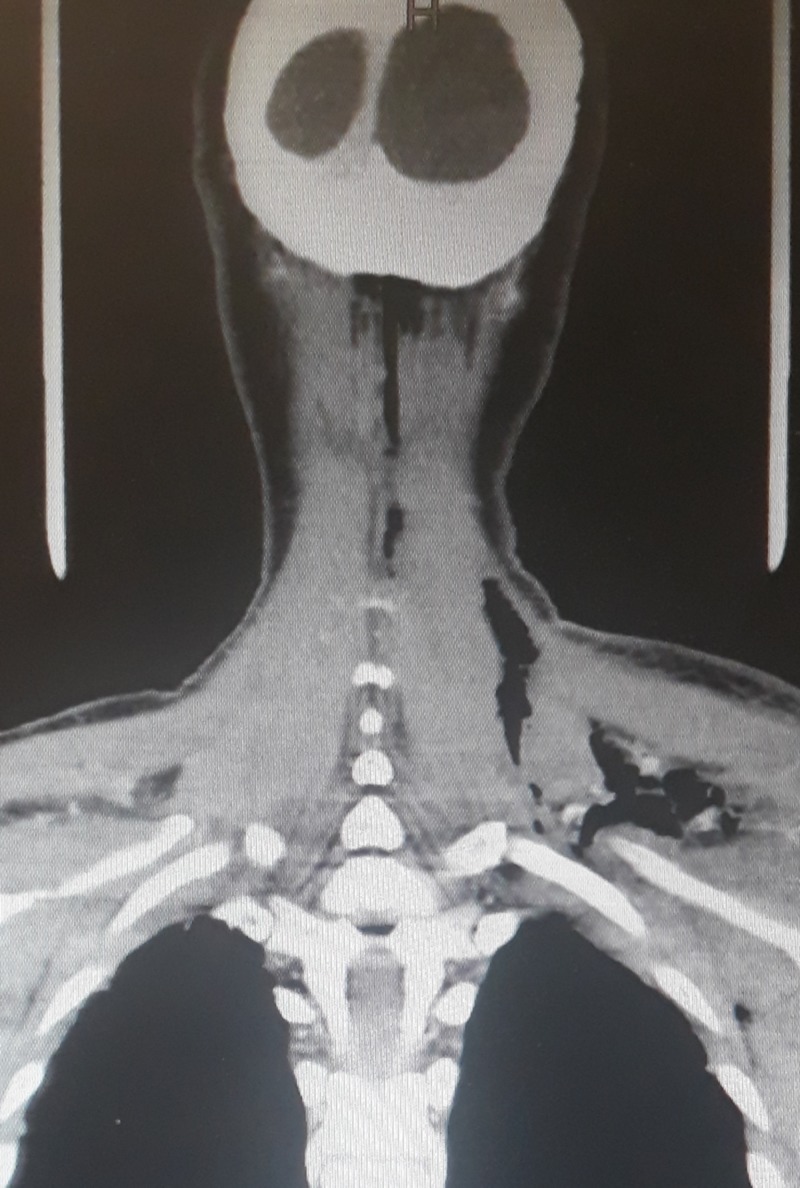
Coronal view of computed tomography of the neck and chest showing subcutaneous air extension into the left supraclavicular area
